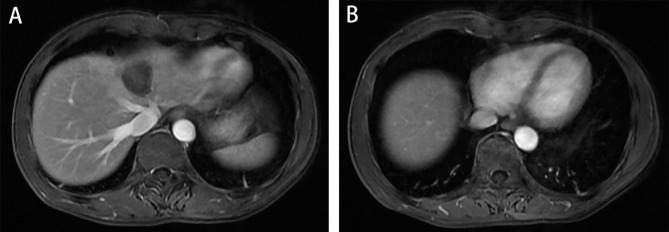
Imaging data from July 29th, 2022 A: MRI showed the S4 microwave ablation zone and left hepatic vein. B: There was no obvious thrombus in the suprahepatic IVC.
